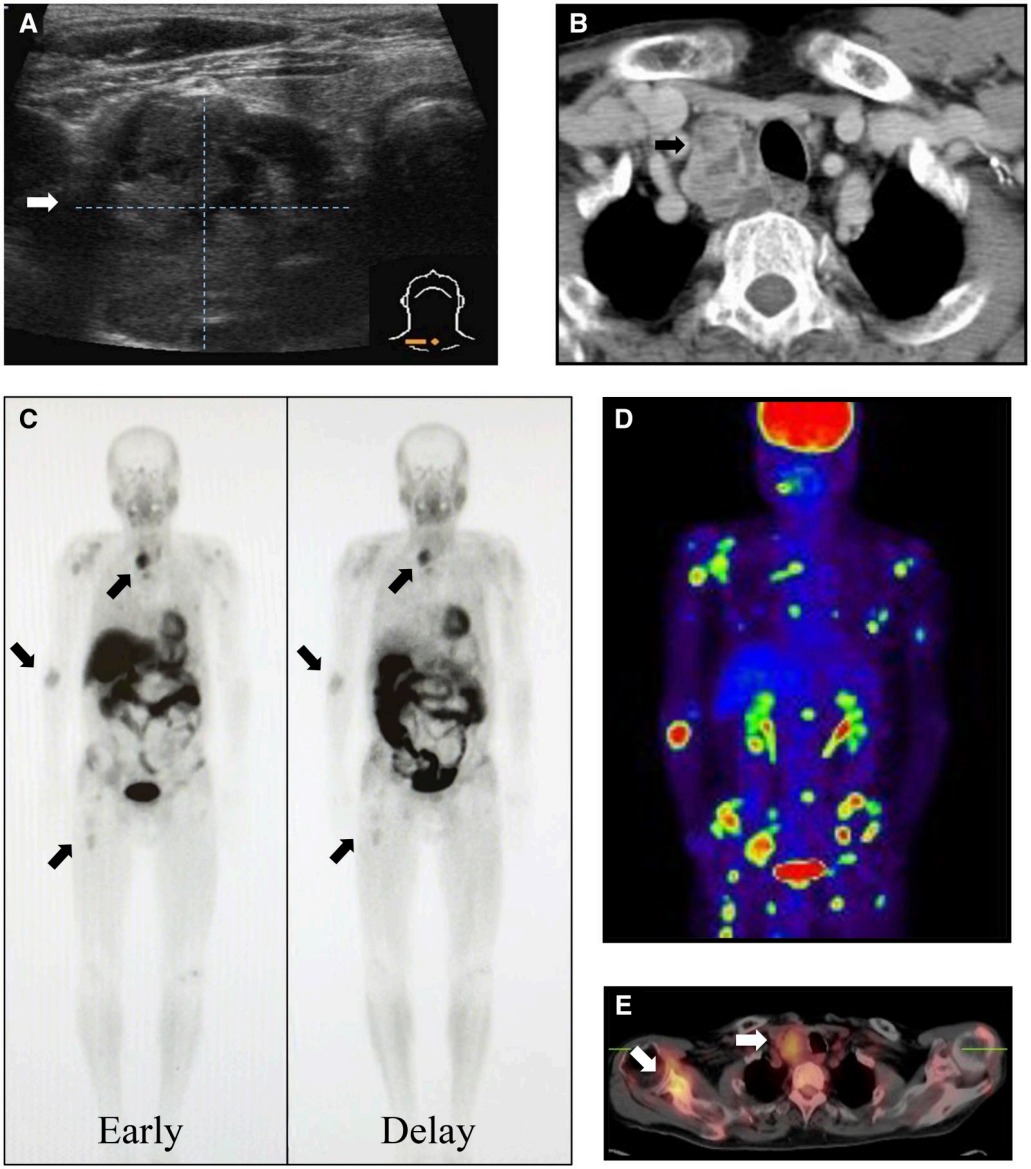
Neck ultrasonography (A) and contrast-enhanced computed tomography (B) show an internally heterogeneous mass at the lower pole of the right lobe of the thyroid gland with an indistinct boundary with the surrounding tissue. 99mTc-MIBI scintigraphy (C) shows abnormal accumulation in the superior mediastinum and multiple bone. 18F-FDG PET/CT also shows a weak accumulation in the superior mediastinum (D) and a strong accumulation of multiple bone (E). 18F-FDG PET/CT = ^18^F-fluorodeoxyglucose-positron emission tomography/computed tomography; 99mTc-MIBI = 99m Tc-methoxy isobutyl isonitrile.
